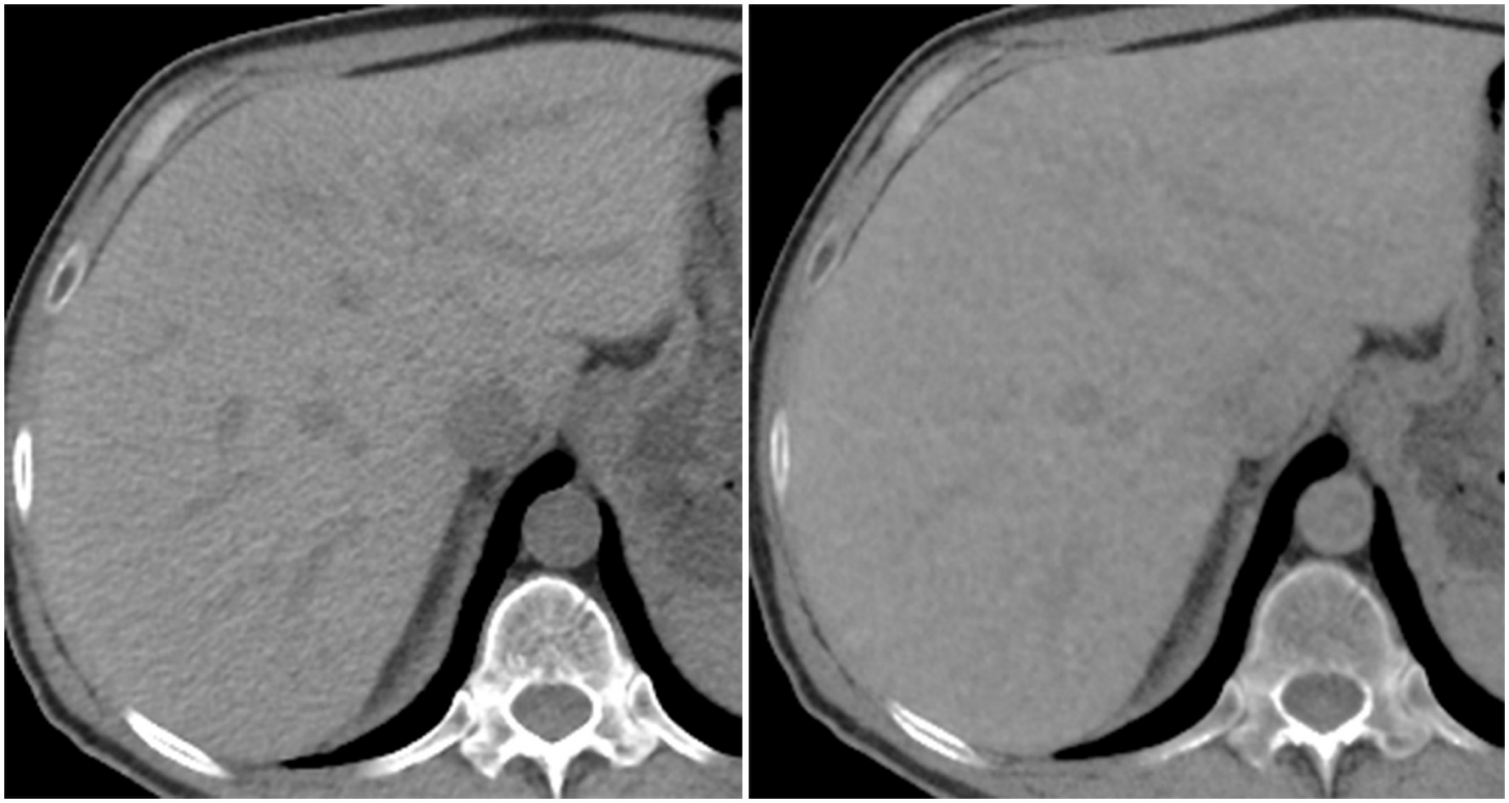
Appearance of liver parenchyma and venous structures on true unenhanced (TUE) image (left) and virtual unenhanced (VUE) image (right). Images are displayed using the same CT window width and window level.
